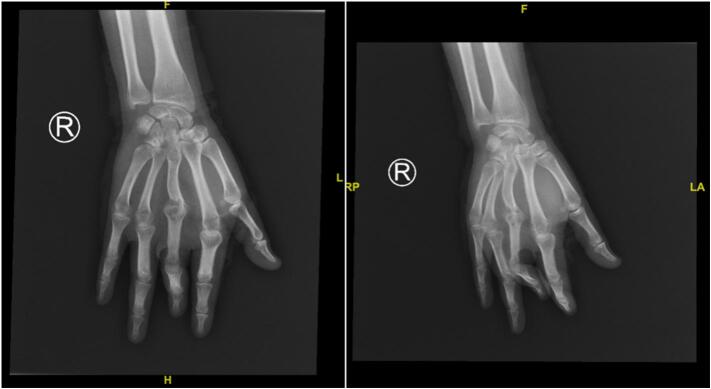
Anteroposterior and Oblique X-Ray of Hand.
